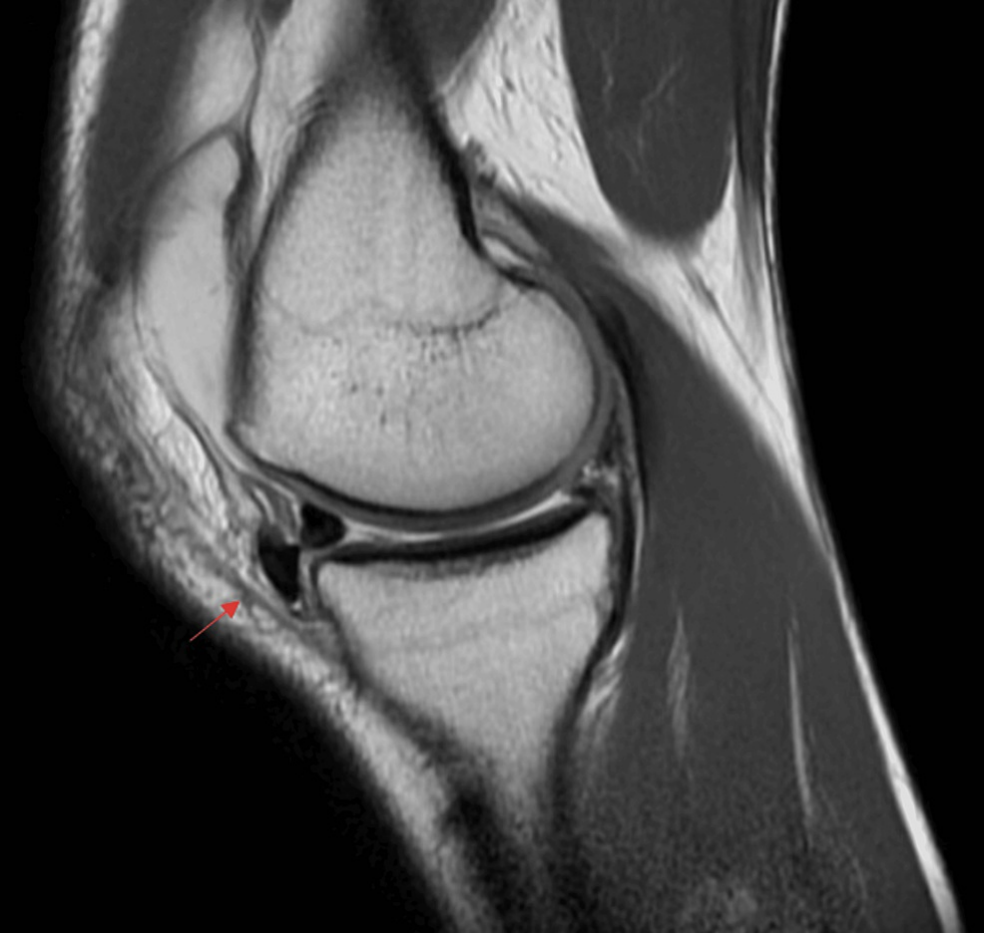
Graft tearing Magnetic resonance imaging showing anterior luxation of the graft (red arrow) resulting in a locked knee. Sagittal view of the left knee, medial meniscus.
